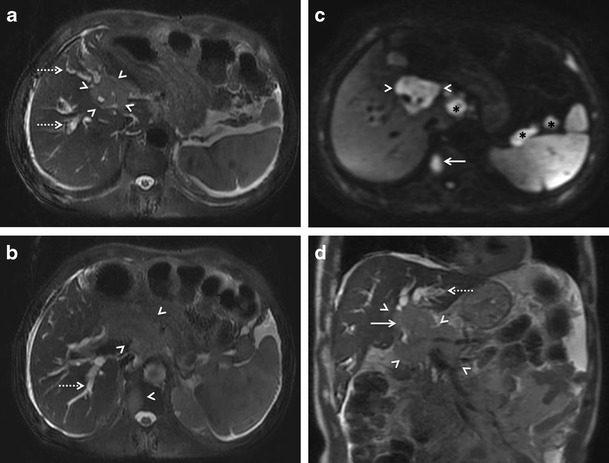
MRI findings in periportal infiltrating variety of hepatic lymphoma in a 60-year-old man. Axial (a and b) and coronal (d) T2-weighted and diffusion weighted (c) MR images demonstrating a mildly hyperintense ill-defined juxta-hilar diffusion restricting mass (arrowheads) completely encasing the common bile duct (solid arrow in d) and vessels at porta with resultant upstream biliary dilatation (interrupted arrows). A vertebral lesion is also seen (solid arrow in b and c) along with perihepatic and splenic hilar lymph nodes (asterisks in c)
